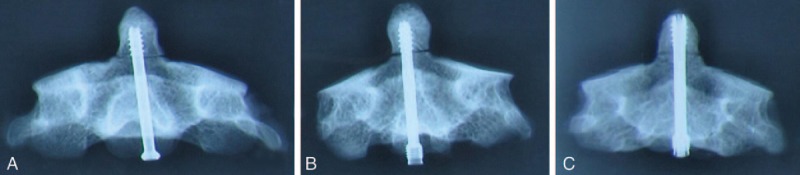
X-ray after screw fixation. (A) Fixed with cannulated lag screw. (B) Fixed with improved Herbert screw. (C) Fixed with expansive double-threaded bi-directional compression screw.
